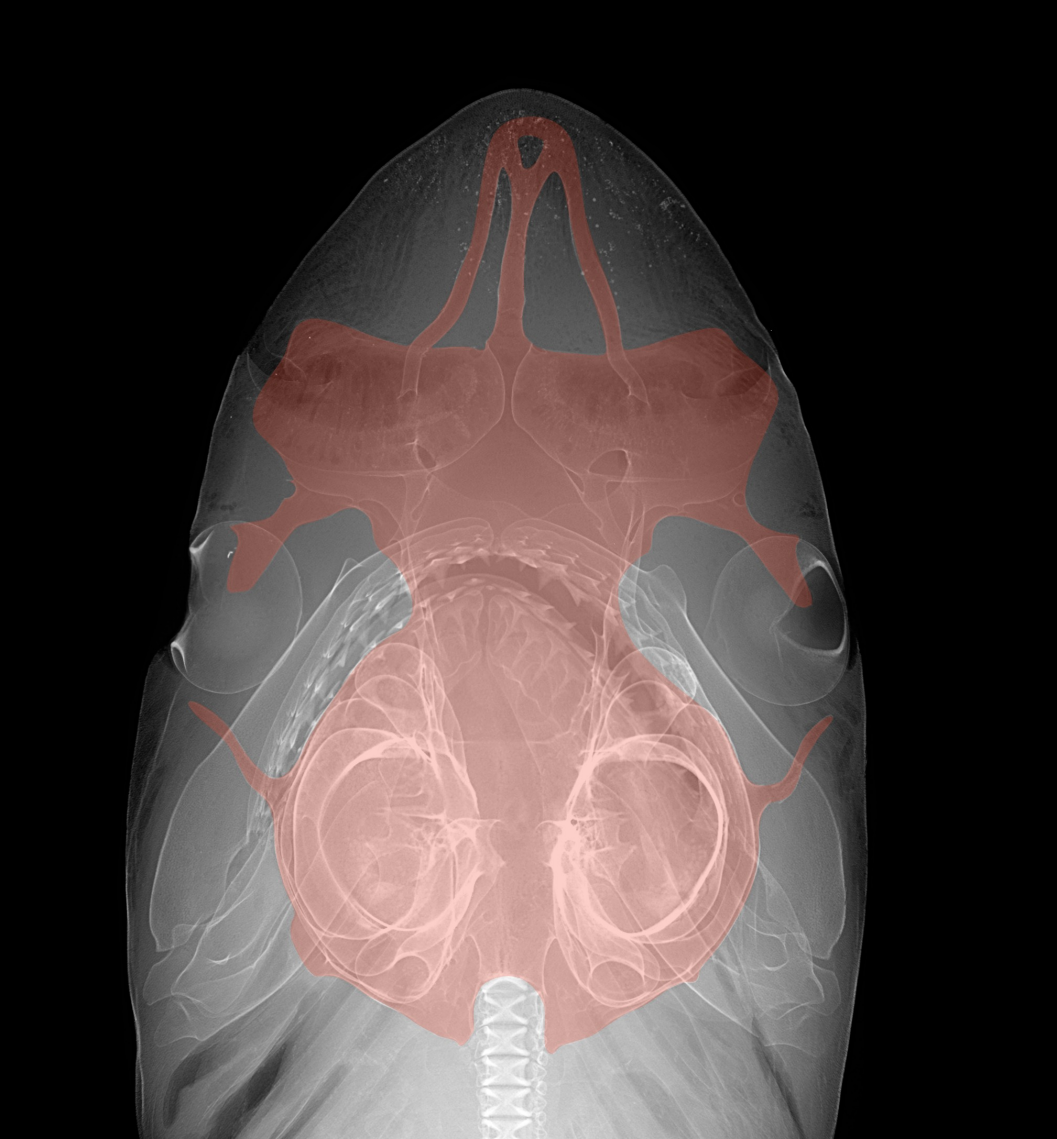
Digital radiograph of the head of *Carcharhinus obsolerus* sp. nov. (ANSP 77121, paratype). 370 mm TL female; chondrocranium highlighted in red.
